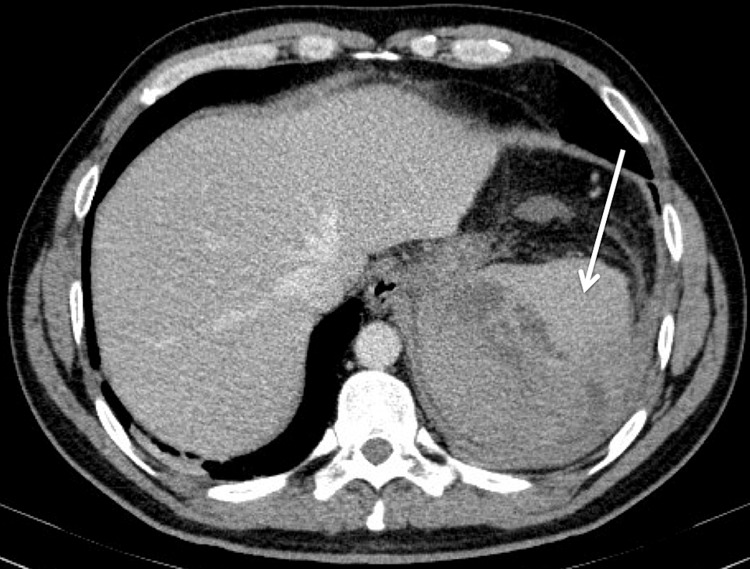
Contrast-enhanced CT scan (axial plane) on admission showing heterogeneous splenomegaly with several hyperdense areas, corresponding to intrasplenic and subcapsular hematomas together with rupture of the splenic capsule.
